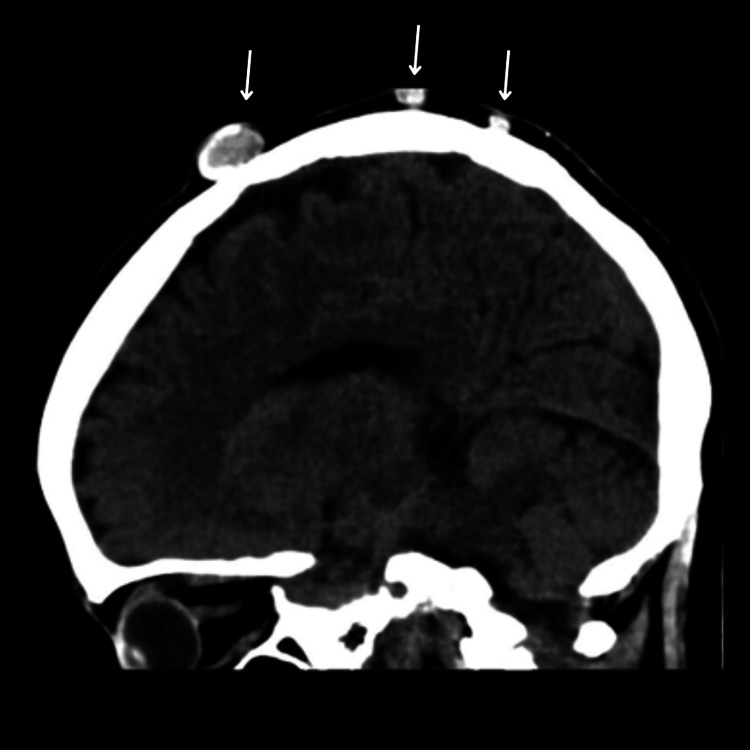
Sagittal view on the noncontrasted CT scan of head Three masses varying in size and depth at the top of the vertex, with partial calcification (arrows) CT: computed tomography
